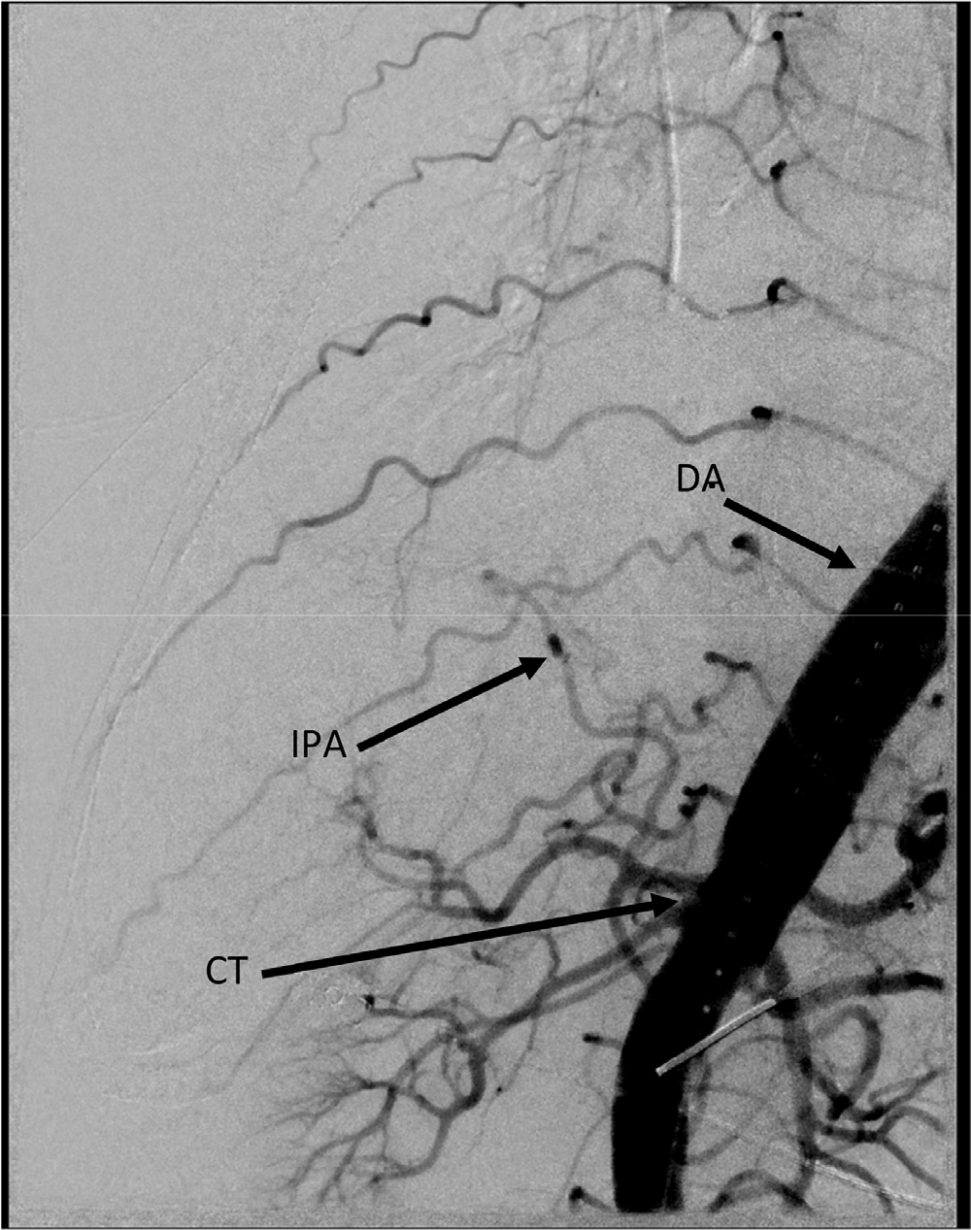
Arteriogram showing right inferior phrenic artery coming off celiac trunk. DA, descening aorta; IPA, inferior phrenic artery; CT, celiac trunk.
